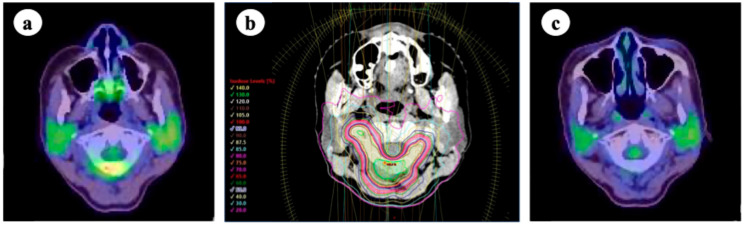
(a) 18-fluoro-deoxyglucose positron emission tomography/computed tomography (FDG-PET/CT) before stereotactic body radiotherapy (SBRT); (b) SBRT targeting the atlas (24 Gy in two fractions, prescribed to 95% of the planning target volume [PTV] and 70% isodose line); (c) FDG-PET/CT 1 year after SBRT. The lesion remained controlled without significant adverse events.
